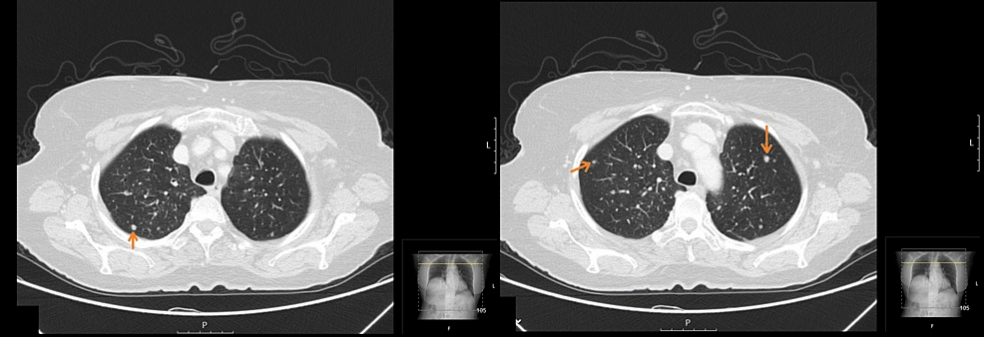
Axial CT scan of the thorax showing multiple bilateral pulmonary nodules (arrows) with less than a centimeter in size CT: computed tomography
